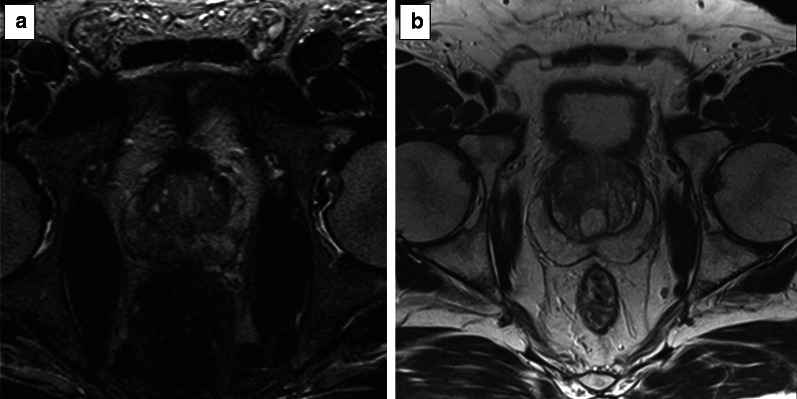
T2-weighted axial images of suboptimal (a) and optimal (b) in-plane resolution
